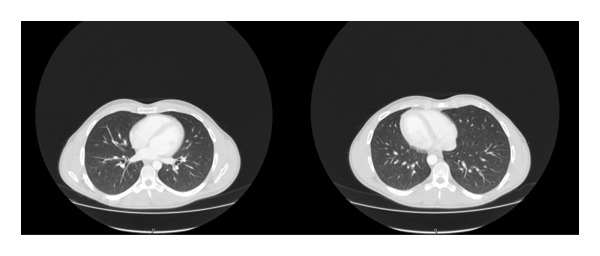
Chest CT imaging demonstrating no pulmonary vascular involvement. Representative axial contrast‐enhanced chest CT images (lung window) obtained to evaluate for pulmonary vascular complications associated with vascular Behçet’s disease. No evidence of pulmonary artery aneurysm, pulmonary artery thrombosis, or other thoracic vascular abnormalities is seen.
